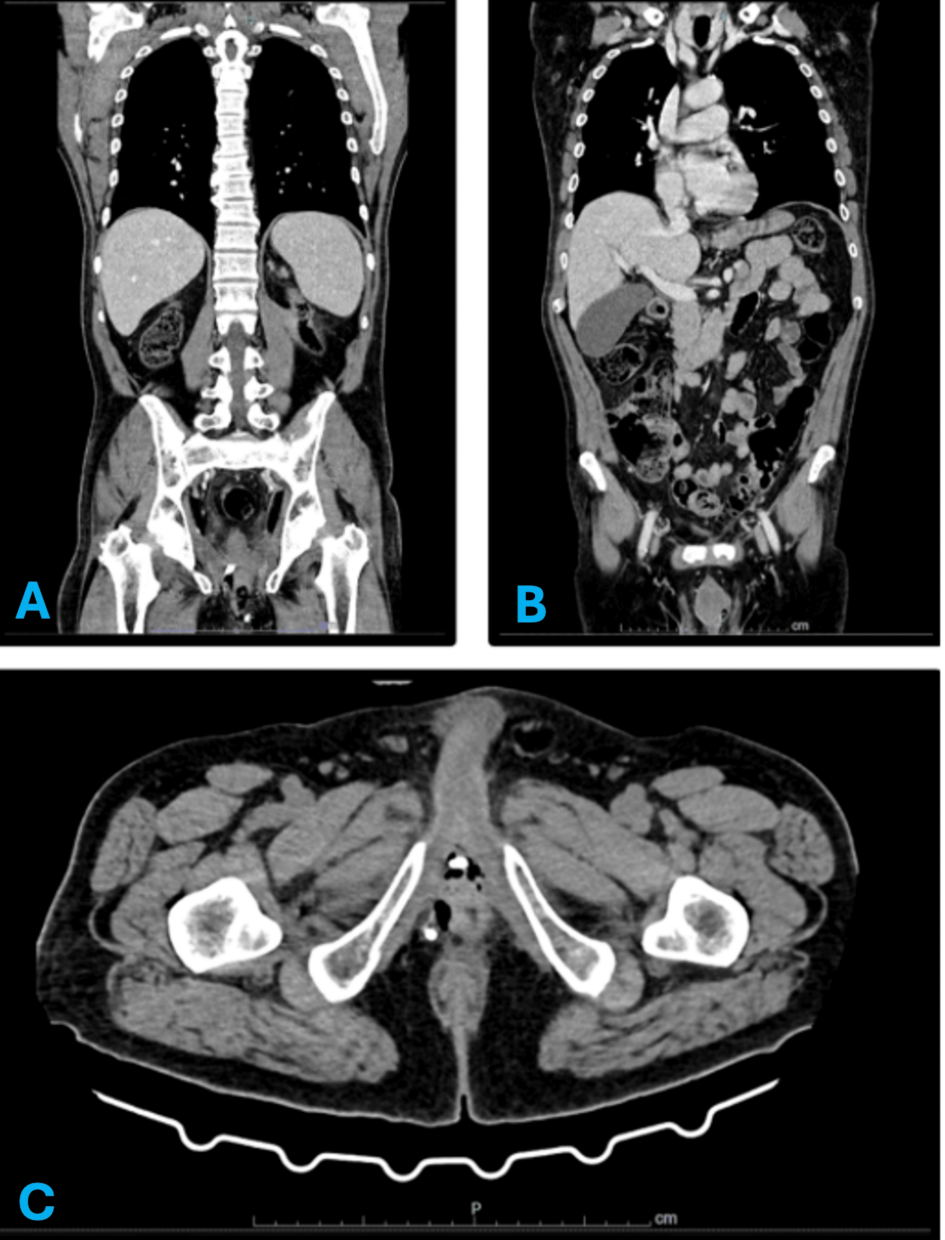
Contrast-enhanced thoraco-abdominal-pelvic CT scan performed in January 2025. (A) Coronal reconstruction demonstrating the upper abdomen without evidence of distant metastatic disease. (B) Coronal reconstruction at a different anatomical level confirming the absence of thoracic or abdominal metastases. (C) Axial pelvic section showing no evidence of local pelvic recurrence or lymph node enlargement.
